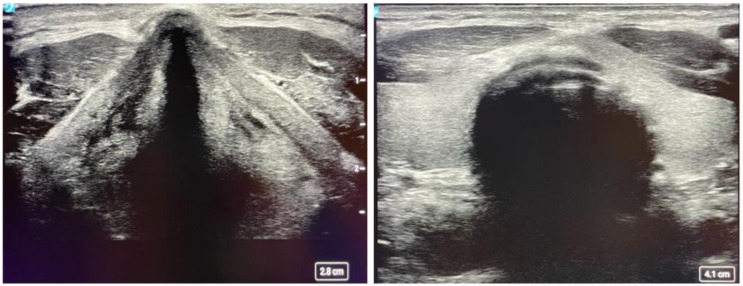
Ultrasound images for percutaneous dilational tracheostomy (PDT). Images are obtained from the level of the cricoid cartilage (Left) and thyroid (Right). The main reason to perform bedside ultrasound prior to PDT is to advance the needle by ultrasound approach and to avoid bleeding risk by identifying central blood vessels, including innominate vein and artery.
